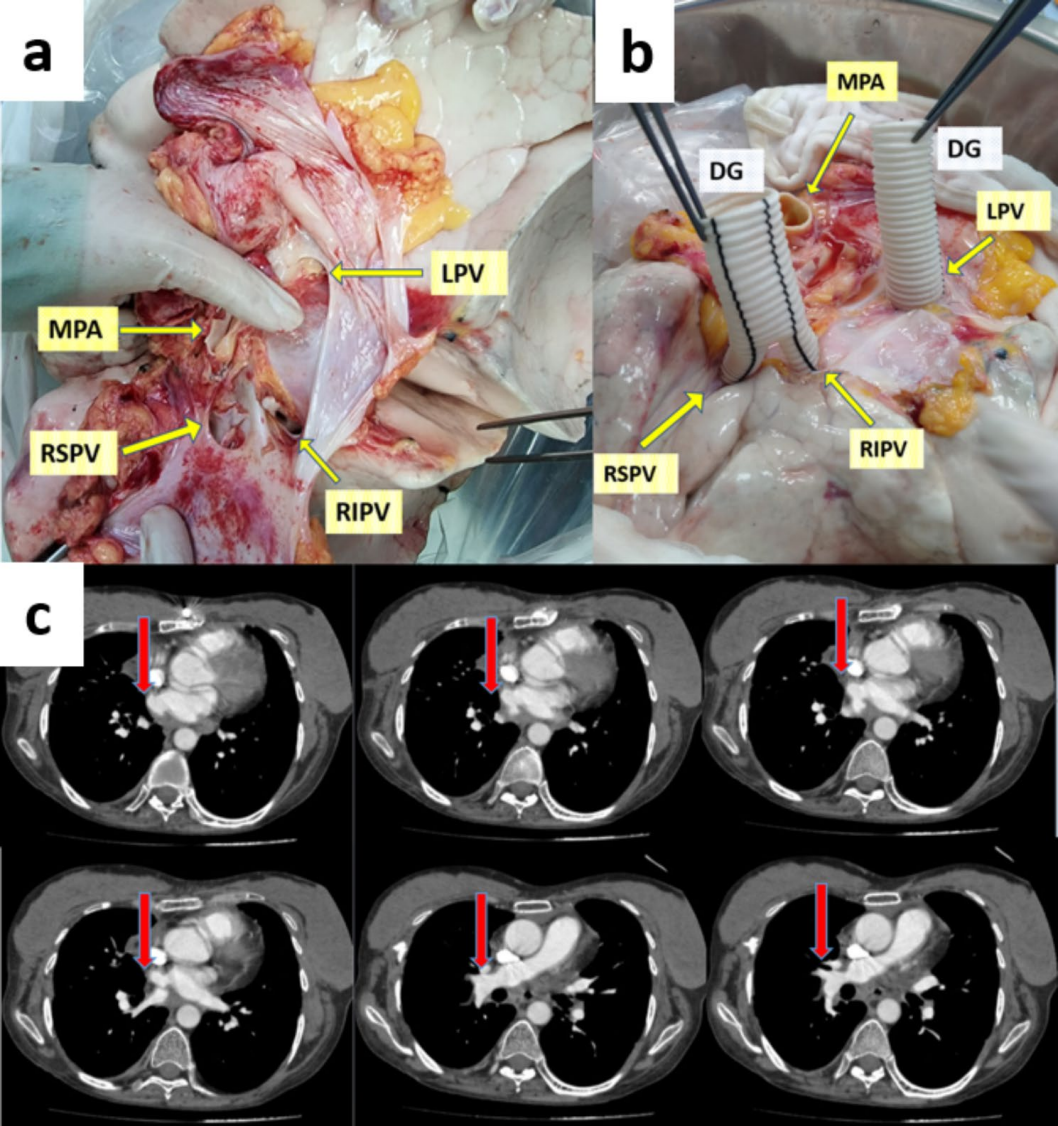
Inadequate cuff, intraoperative photographs showing reconstruction with dacron mash, and postoperative Pulmonary Computed Tomography Angiography images. (a) Insufficient left atrial cuff: RIPV: Right inferior pulmonary vein, LPV: Left pulmonary vein, MPA: main pulmonary artery, RSPV: right superior pulmonary vein. (b) Left atrium repaired with Dacron Mash, RIPV: right inferior pulmonary vein, PV: left pulmonary vein, MPA: main pulmonary artery, RSPV: right superior pulmonary vein, DG: dacron graft. (c) Anastomosis line (arrow) in the third month postoperative Pulmonary Computed Tomography Angiography
